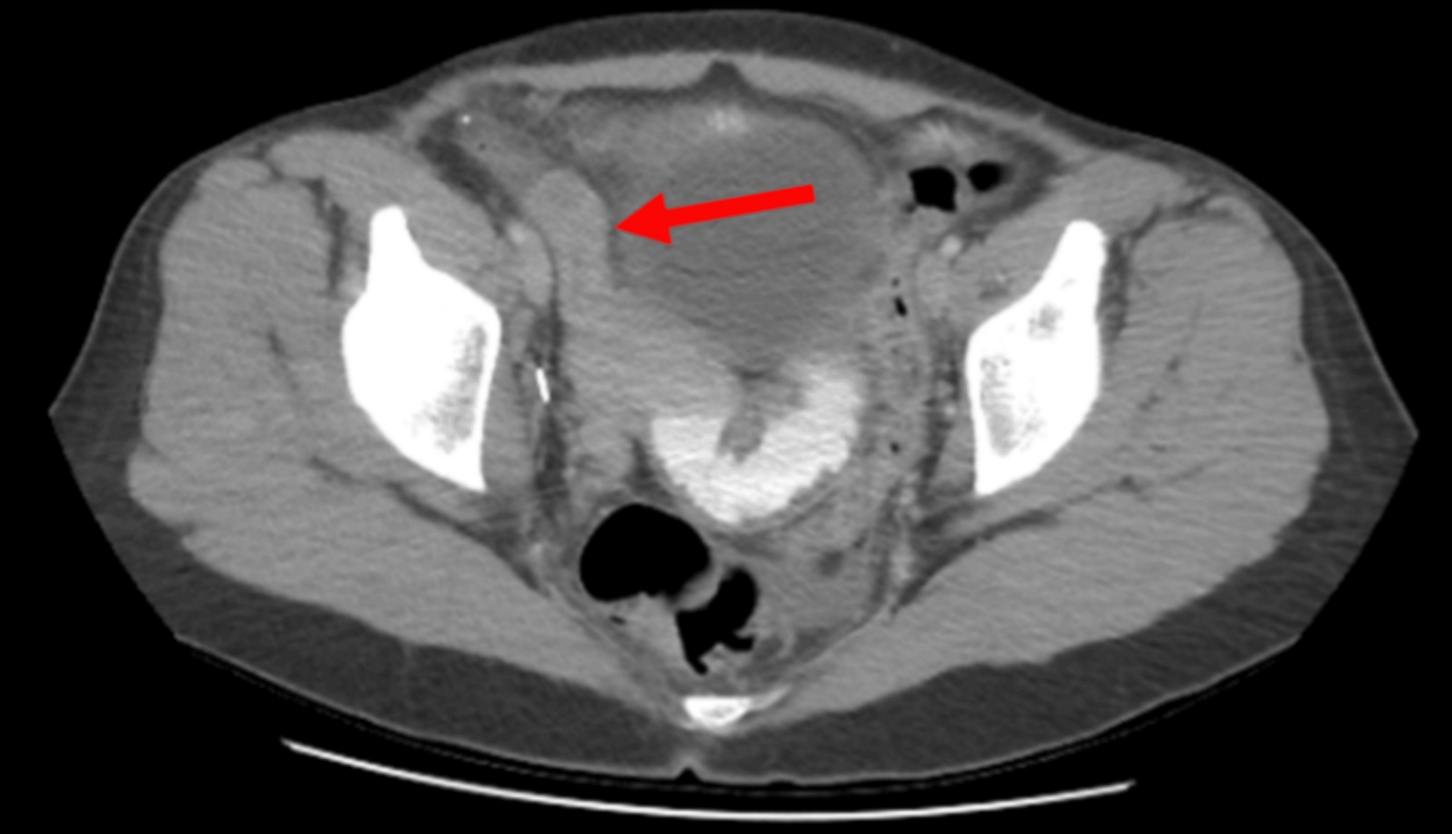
Intramural edema. The patient underwent PET-CT imaging with injection of 18F-fluorodeoxyglucose, which demonstrated wall thickening and intramural edema within the terminal ileum (arrow). The recurrent metastatic soft tissue mass in the right pelvis extends into two segments of the terminal ileum and abuts the right lateral wall of the rectosigmoid junction.
